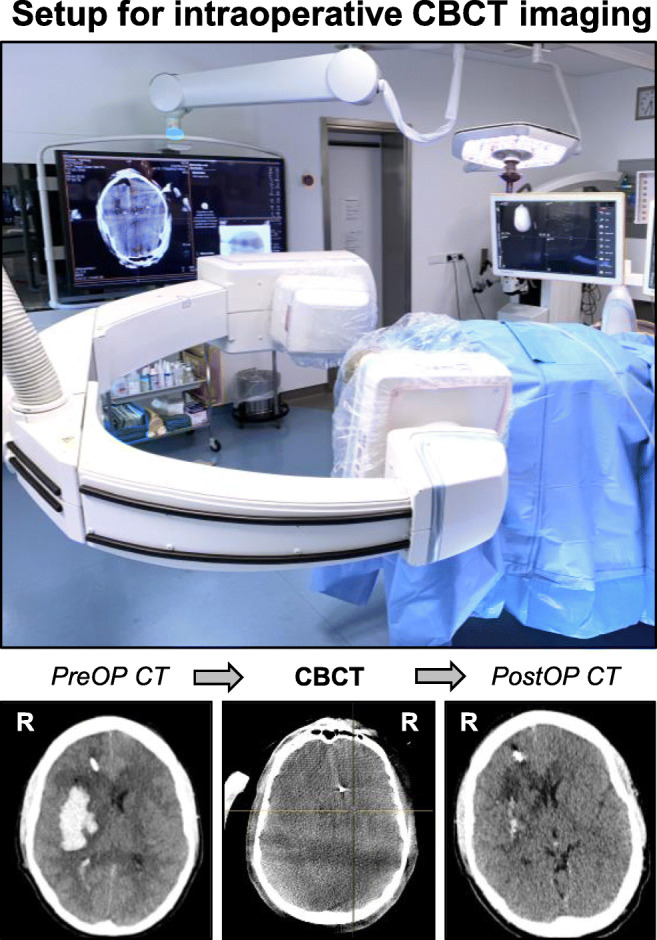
Image quality of intraoperative CBCT versus conventional CT imaging. The upper panel shows the setup for intraoperative CBCT scanning after hematoma evacuation. In contrast to iCT, the CBCT flat panel detector rotates around the patient and requires draping to maintain sterility during the scan. Below, the imaging sequence in patient 18 shows sufficient CBCT image quality for reliable intraoperative hematoma assessment compared to conventional pre- and postoperative CT imaging, despite lower signal-to-noise ratio. R, right
